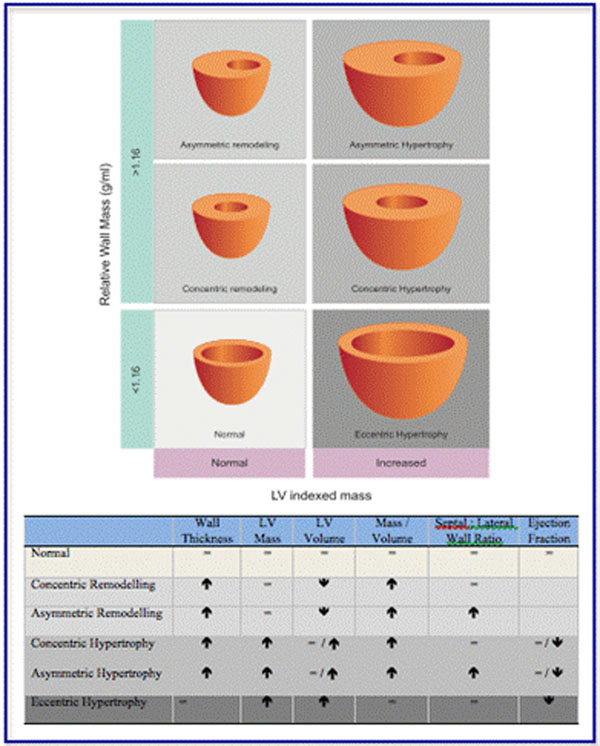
Cardiac magnetic resonance imaging definitions of LV hypertrophy and remodelling
